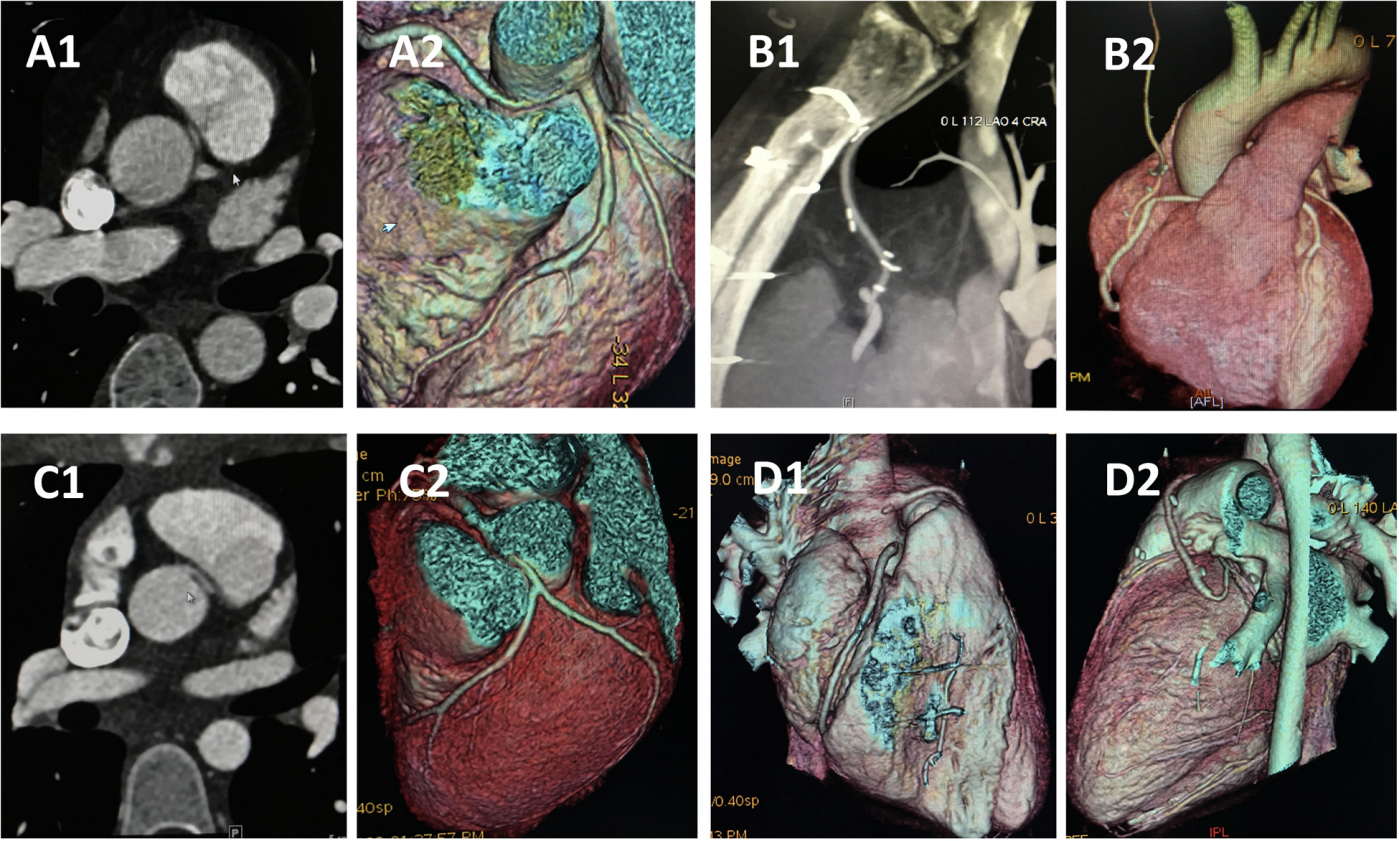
CCTA of case 5 and case 8 underwent CABG. Case 8: (A) Preoperative images showing anomalous right coronary artery arising from the left sinus. (B) Follow-up images showing right internal mammary artery grafts to right main coronary artery without visible stenosis. Case 5: (C) Preoperative images showing anomalous left coronary artery arising from the right sinus. (D) Follow-up images demonstrated that the left internal mammary artery was obstructed 14 monthes after operation. CCTA, coronary computed tomography angiography; CABG, coronary artery bypass graft.
